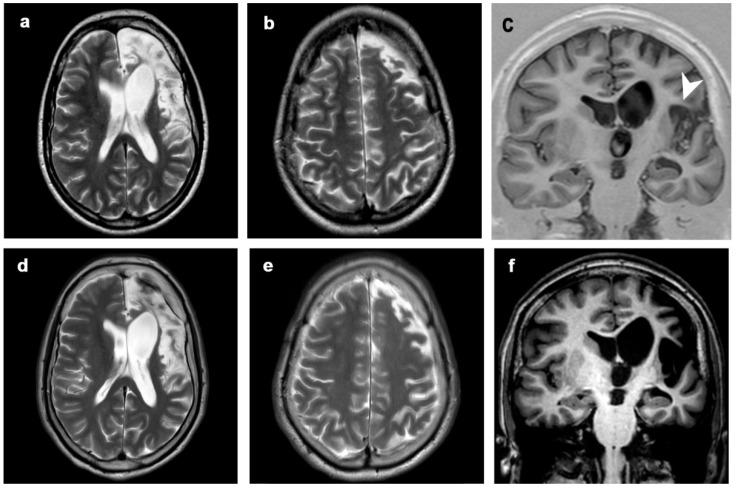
Patient 2, progression of the disease in 21 years. Axial (a,b,d,e) T2-weighted images, coronal T1-inversion recovery (c) and T1-weighted image (f) at (a–c) 5 years (first MRI scan) and (d–f) 21 years from clinical onset (last MRI scan). The disease showed mild progression over time, involving left perisylvian region (including frontal, insular and temporal structures, arrowhead). Clinical symptoms progressed from seizures at onset to seizures, focal deficits, EPC, cognitive and behavioral disorders.
